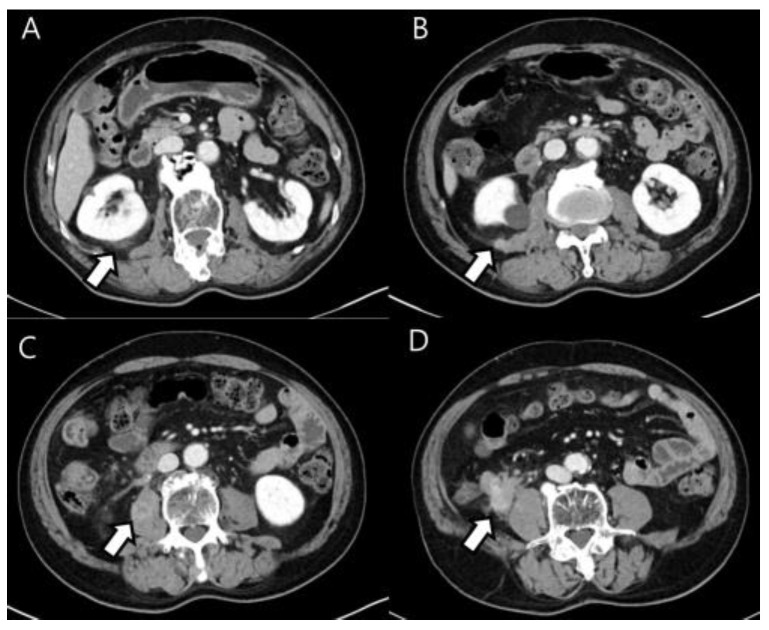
Axial, intravenous, contrast-enhanced CT scan of the abdomen (A–D) reveals an infiltrative-enhancing soft tissue mass in the terminal ileum. It also shows several enhanced soft tissue nodules in the right Gerota’s fascia, transversalis fascia, posterior pararenal fascia and right psoas muscle.
